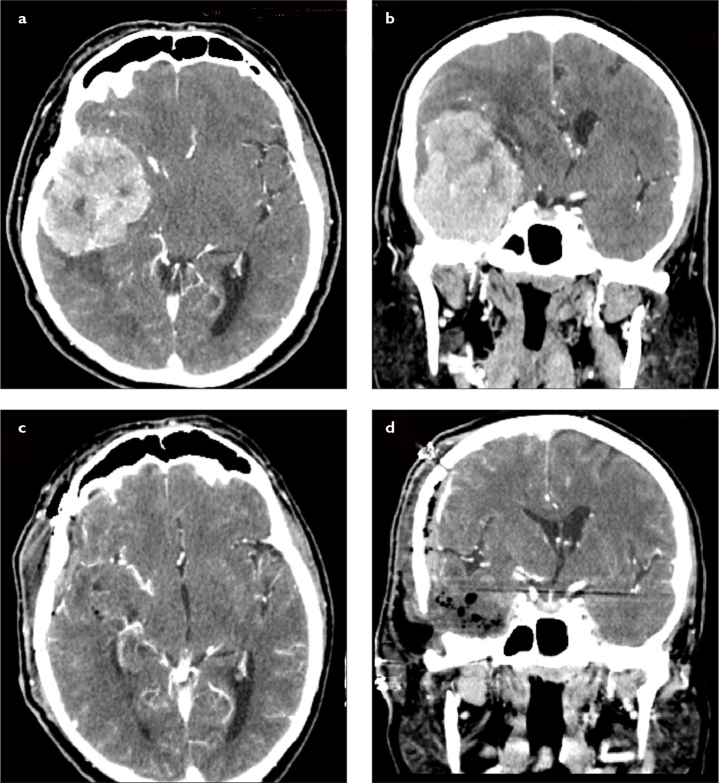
(a) Preoperative axial and (b) coronal CT scans (with contrast) of a patient with giant right temporal fossa meningioma originating from the skull base dura mater. There was a significant midline shift and brain edema. The patient underwent gross total resection using right temporal craniotomy. (c) Postoperative axial and (d) coronal CT scans (with contrast) confirmed gross total resection. The shift was improved and the resolution of brain edema was obvious. CT, computed tomography.
